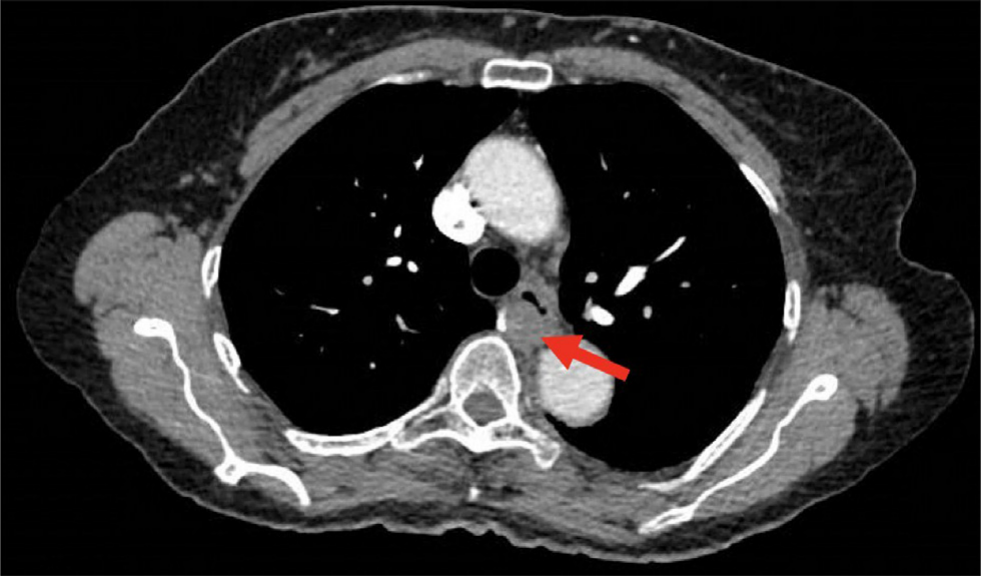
CT scan of chest demonstrating mass in posterior esophageal wall at the level of T5-T7.
